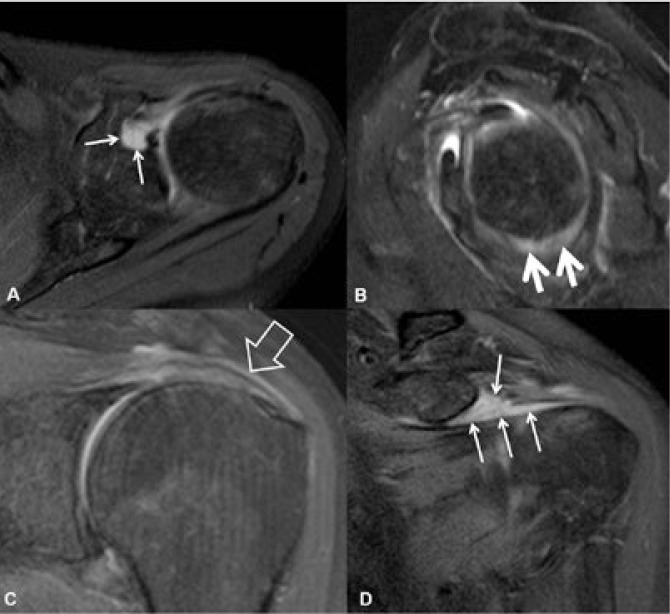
A 62-year-old female patient with 2-month pain in the left shoulder. Fat suppressed PD-w MR images in the axial (a), oblique sagittal (b), and oblique coronal (c,d) planes, show the abnormal signal in the rotator cuff interval (thin arrows a,d), and in the axillary recess (thick arrows, b). The abnormal signal in the supraspinatus tendon (open arrow, c), suggests degenerative tendinopathy.
